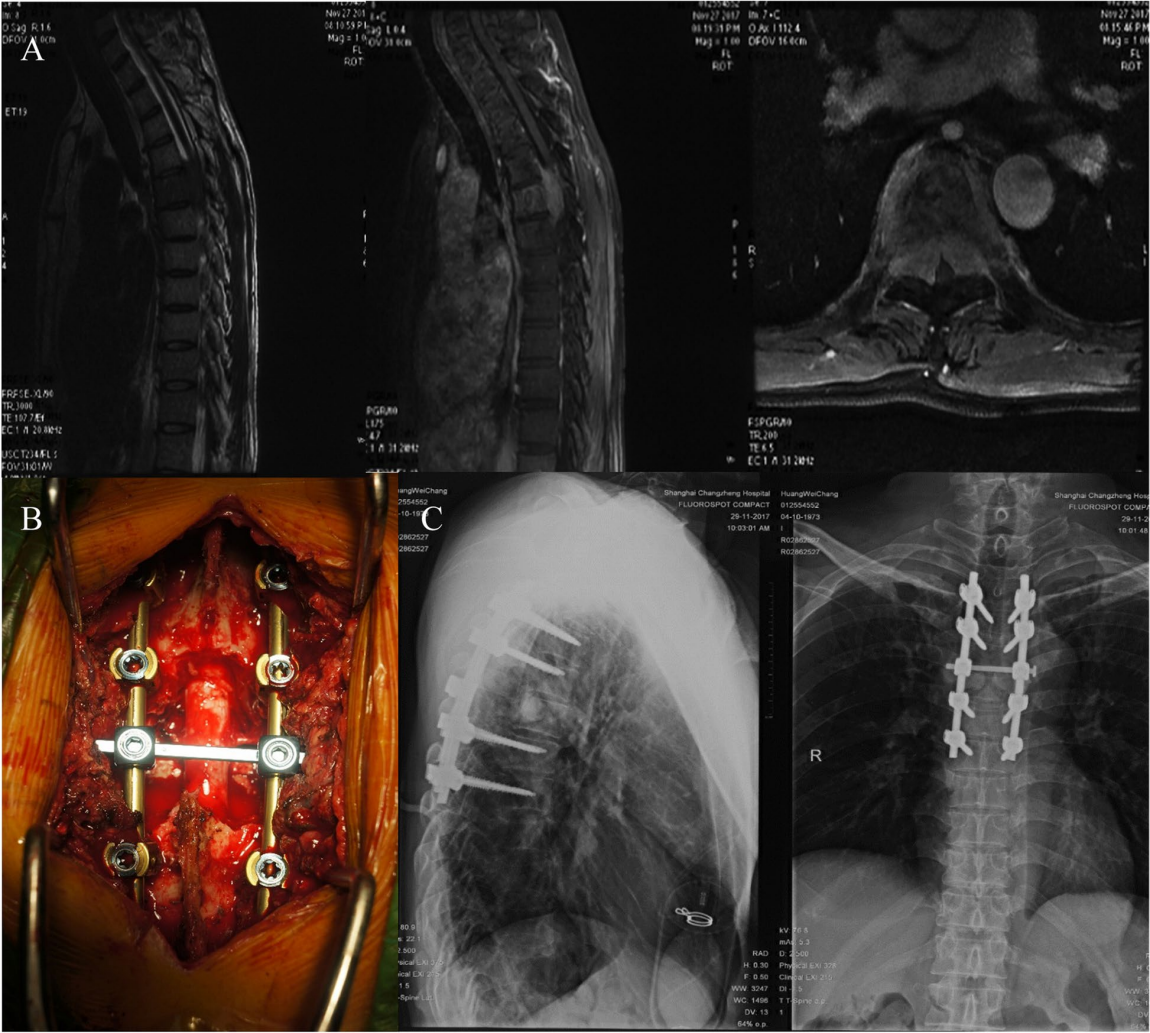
Images of a 44-year-old man with T5 metastasis from esophageal cancer who underwent palliative surgery. A MR images showing the vertebral tumor extending into the spinal canal with dural sac compression. B Intraoperative photographs displaying the PSRS performed. C Postoperative X-ray
